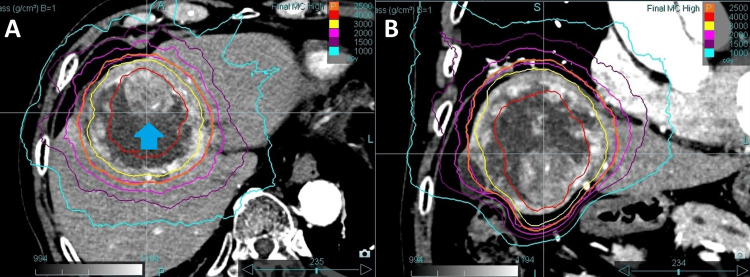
Dose distribution in second-stage SBRT Contrast-enhanced CT showing dose distribution for the second-stage SBRT in (A) axial and (B) coronal views. A total dose of 25 Gy was delivered in five fractions. Isodose lines, from outermost to innermost, represent 10 Gy, 15 Gy, 20 Gy, 25 Gy, 30 Gy, and 40 Gy, respectively. The arrow indicates the recurrently enlarged solid tumor component. SBRT, stereotactic body radiotherapy
